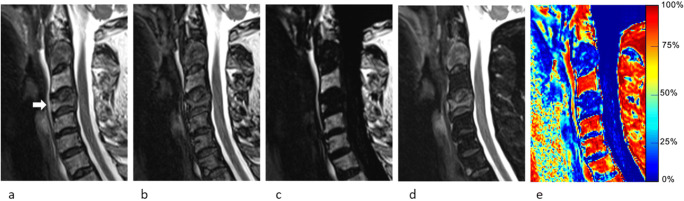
71-year-old man with a malignant vertebral compression fracture (VCF) at C4 level (arrow). Sagittal cervical spine MR images generated from a signal FSE T2-weighted Dixon sequence include (a) in-phase, (b) out-of-phase, (c) fat-only, (d) water-only images, and (e) fat fraction (FF) map. While readers all agreed on the malignant nature of the VCF, quantitative analysis was falsely negative for signal drop with both ROIs using the cutoffs determined in this study (signal drop, FF calculated from water-only and fat-only images, and FF from maps were ROI1: 25.8%/ROI2: 25.4%; ROI1: 14.3%/ROI2: 1.7%; and ROI1: 12.2%/ROI2: 10.9%, respectively)
